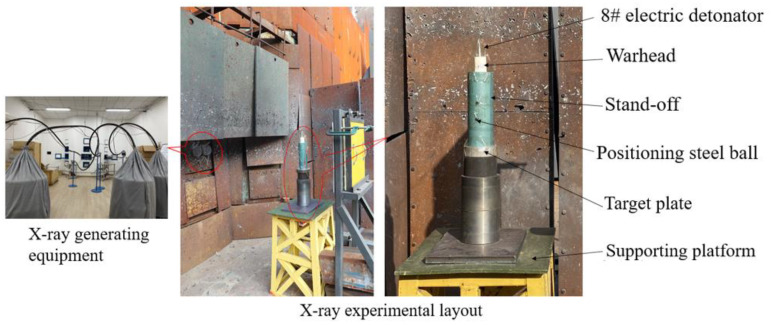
Jet X-ray shooting equipment and test site layout.
